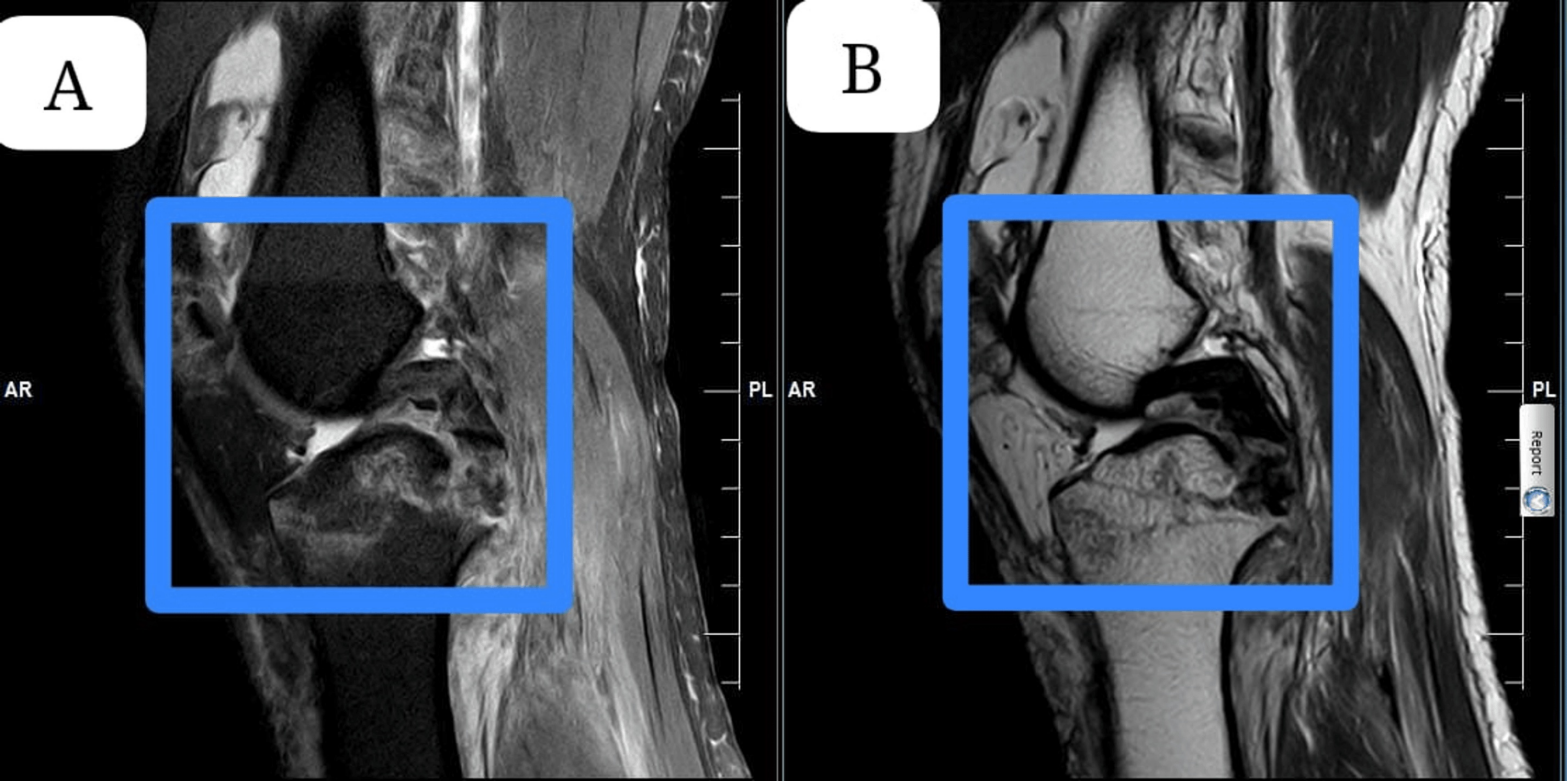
The MRI scans show a posterior cruciate ligament (PCL) injury. Panel A-B: Preoperative MRI scan of the knee showing the affected area. The highlighted region indicates abnormalities in the joint structure.
